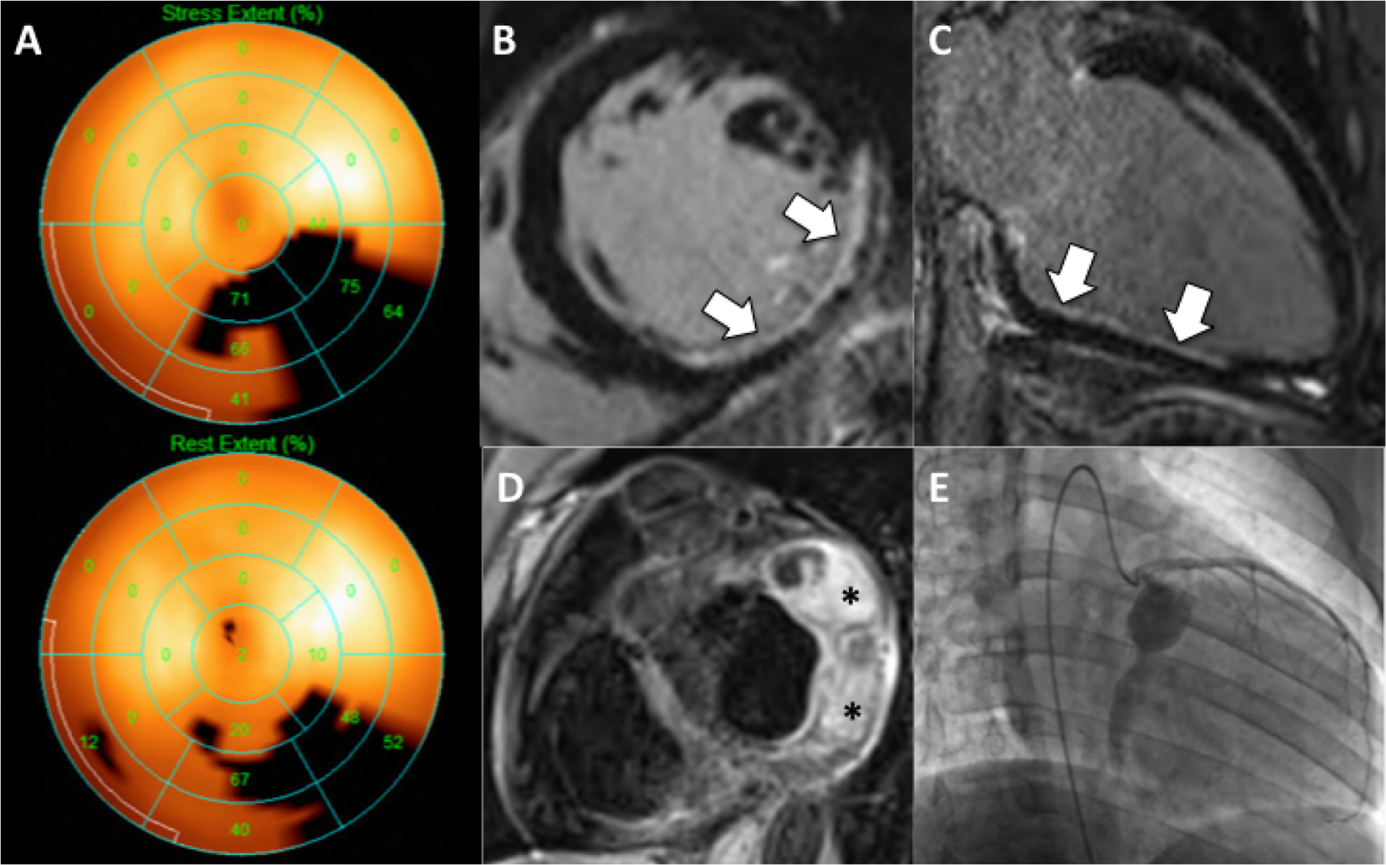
Circumflex territory infarct. A) Nuclear SPECT perfusion study demonstrating a largely fixed infero-lateral defect on polar map. B,C) Late gadolinium enhancement reveals a non-transmural scar (white arrows) in the same area. D) Black-blood CMR shows circumflex aneurysms with thrombus (asterisks). E) Angiogram confirms Cx aneurysms with poor distal flow.
